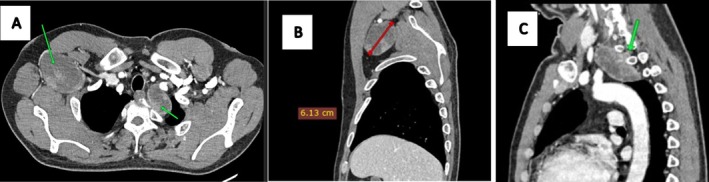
Contrasted CT scans of an axial slice (A), and sagittal reformats (B, C) showing well‐demarcated expansile heterogeneously enhancing soft tissue‐density masses in the right axilla and supero‐posterior mediastinum (green arrows) with mass effect to the surrounding structures, measuring (6.8 × 6.1 × 4.2) cm; the mediastinal mass has a spinal connection as seen by the arrow in C, a sagittal image giving it a characteristic ‘dumb‐bell’ shape.
